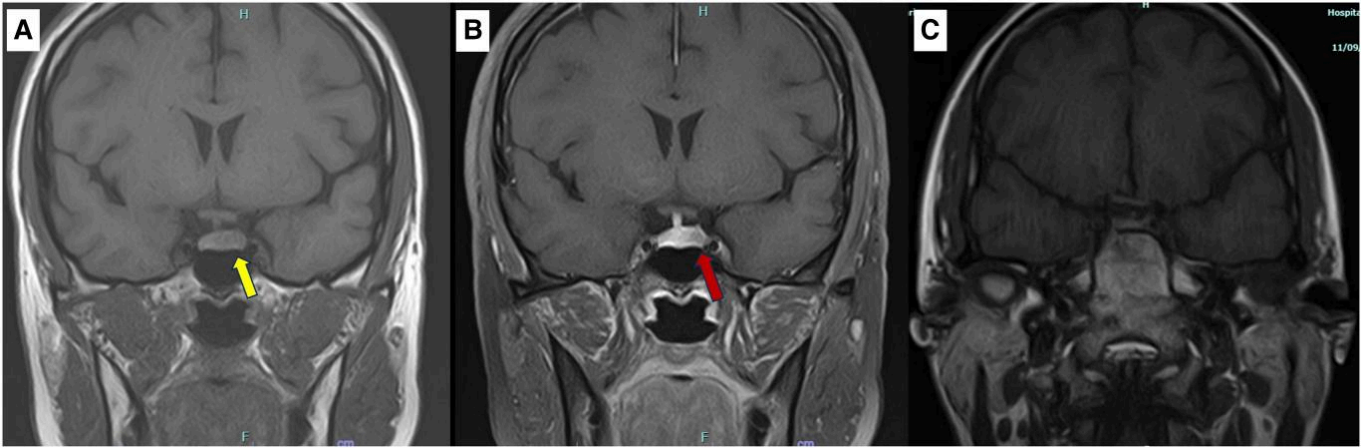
Coronal T1-weighted MRI pituitary at diagnosis and after transsphenoidal surgery. (A) A hypointense lesion on the T1-weighted image in the left anterior pituitary region, measuring 0.6 × 0.5 × 0.5 cm (yellow arrow). (B) The lesion exhibited minimally delayed enhancement following gadolinium contrast administration (red arrow). (C) Follow-up MRI 6 months after transsphenoidal surgery. Abbreviation: MRI, magnetic resonance imaging.
